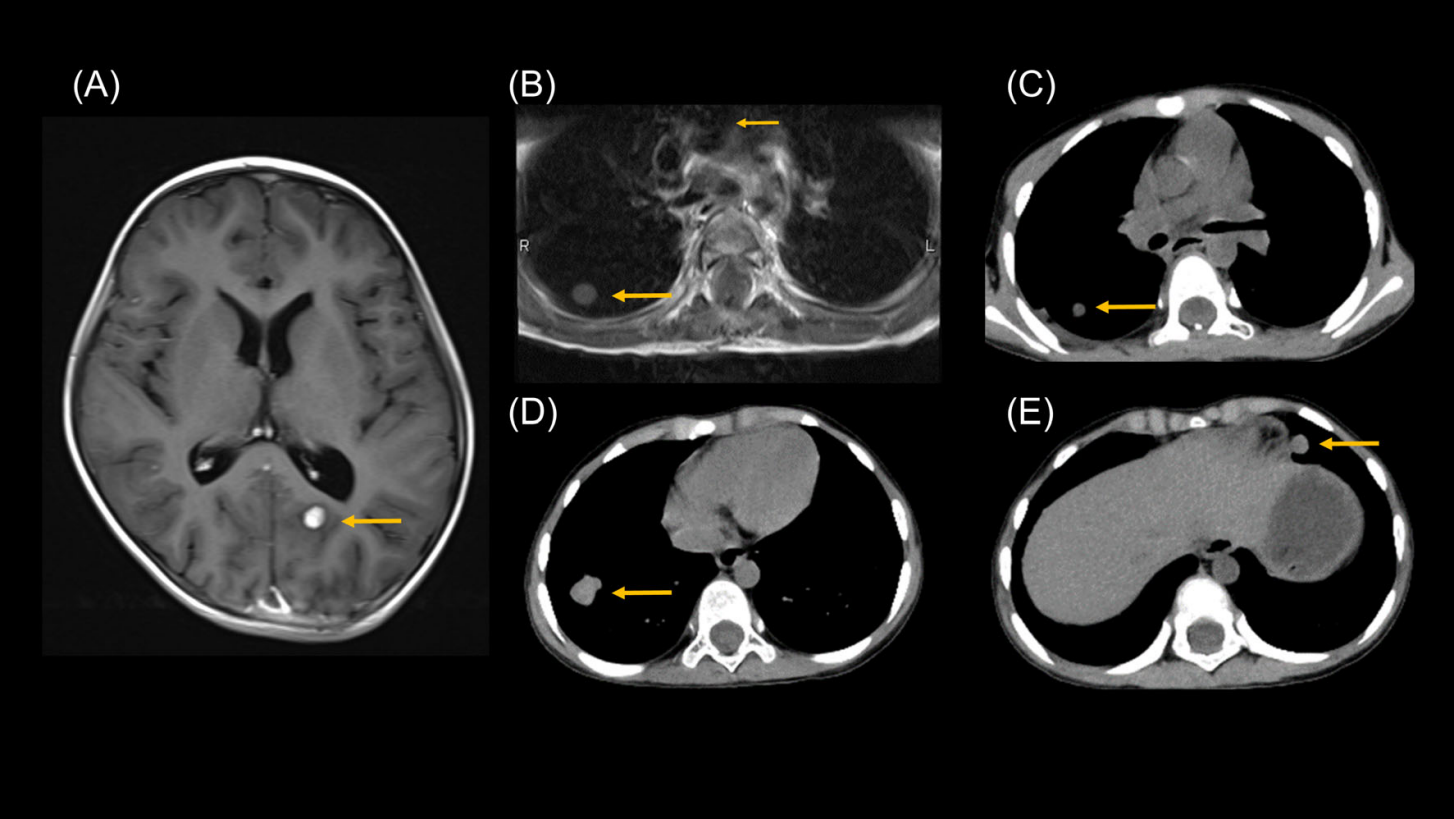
After irradiating the mass in Figure 3, Gd‐T1w MRI showed a 6 mm contrast‐enhanced nodule in the left parieto‐occipital lobe (A). Spinal MRI incidentally revealing a lung lesion (B). Whole‐body computed tomography (CT) was performed to search for metastases, and multiple masses were found in the lungs (C)–(E). There were no lesions in other body parts, including the kidneys and liver.
